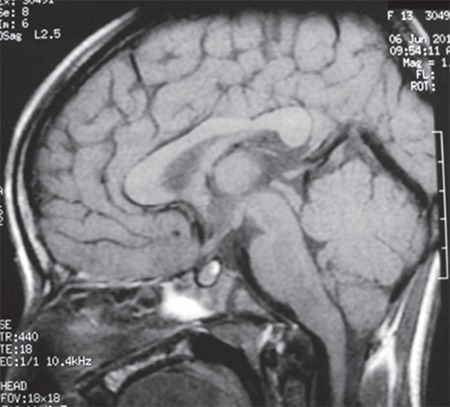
Magnetic resonance imaging of brain (T1W) showing normal pituitary and suprasellar region with normal pineal gland
